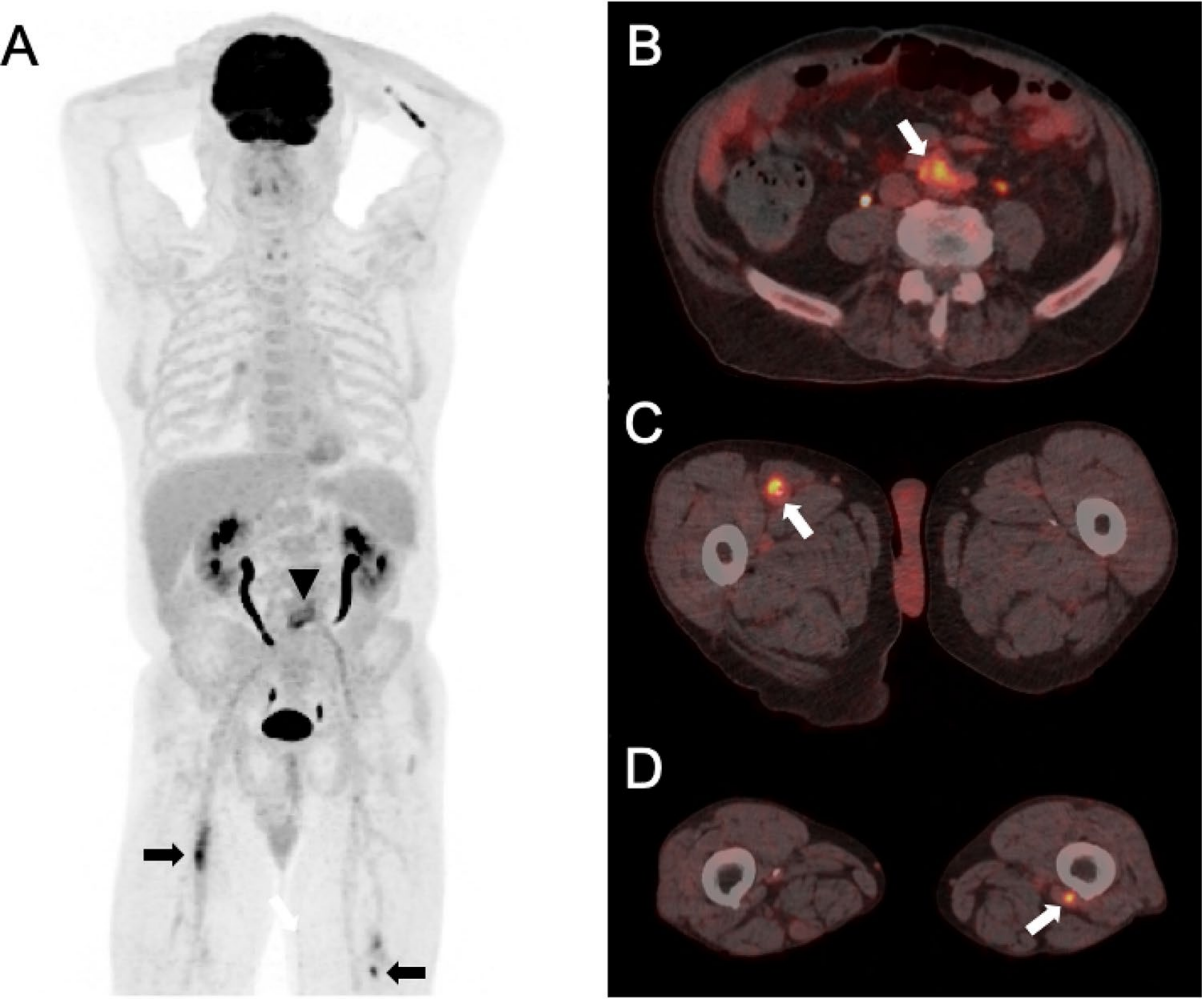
A 66-year old male patient was referred to PET/CT for follow-up of a vascular graft infection with *P. aeruginosa*. PET/CT [maximum intensity reconstructions of PET (A) and fused PET/CT images (B–D)] showed intense focal FDG uptake of the aortobifemoral graft (black arrow head in A, white arrow in B), suggestive for persistent infection. Subsequently, the aortobifemoral graft was reconstructed in situ with a SilverGuard Dacron graft. An unknown and potentially relevant incidental finding was detected in the upper thighs with intense focal FDG uptake, in line with septic emboli (black arrows in A, white arrow in C and D). The incidental PET/CT finding was rated not to have impact on patient management, since no direct action was initiated with regard to the potential septic emboli in the upper thighs.
